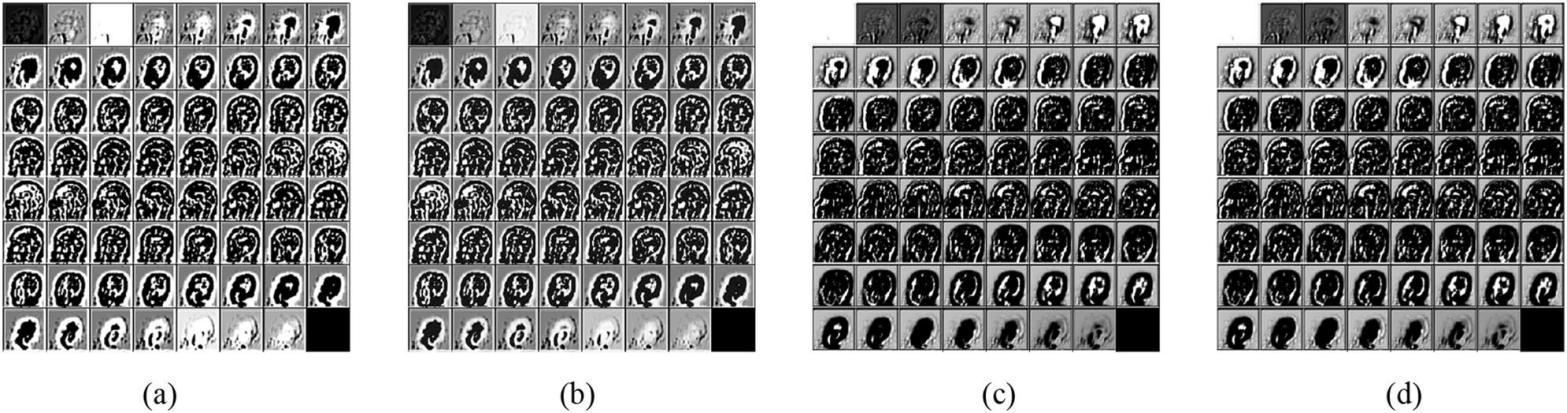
Comparison of activation using different functions for a sample MRI observed in 2nd activation layer (22nd of 64 channels) corresponding to 63 × 63 × 63 image as a montage from (a) Input (b) Output using SGT (c) Output using ReLU (d) Output using Leaky-ReLU. It can be observed that the output from the SGT, Fig. 1b), has well preserved the feature attribute present in the first three and last few slices in comparison to ReLU (c) and Leaky ReLU (d) where (a) is the input feature matrix.
